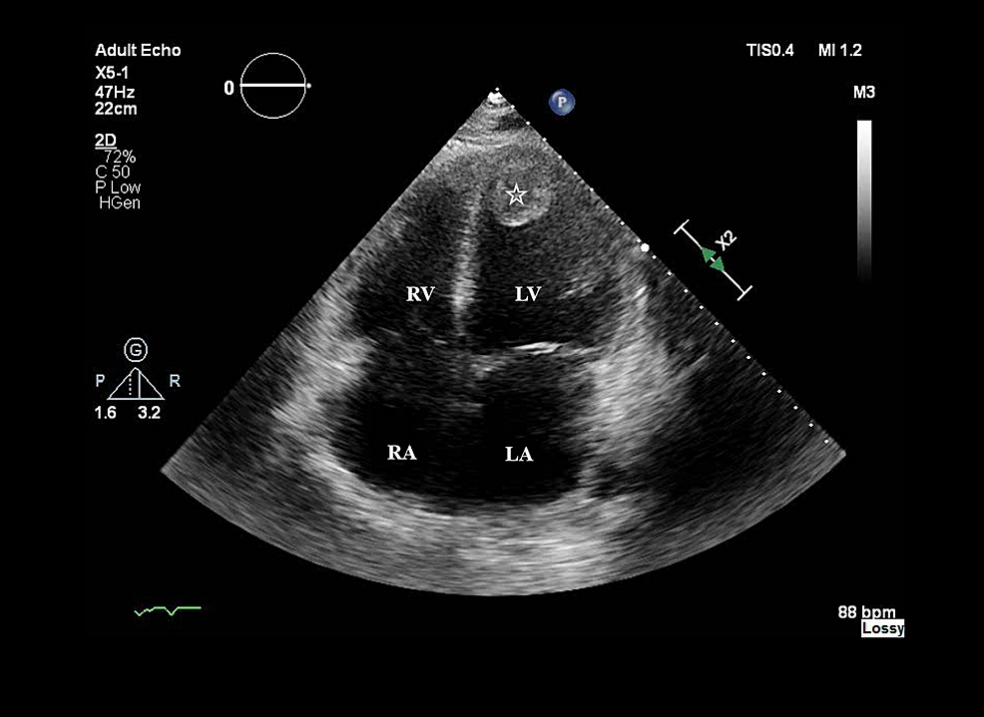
TTE apical view reveals the presence of an organized mass of 3 cm × 2.7 cm (White star) attached to the left ventricular apex. TTE: transthoracic echocardiogram
